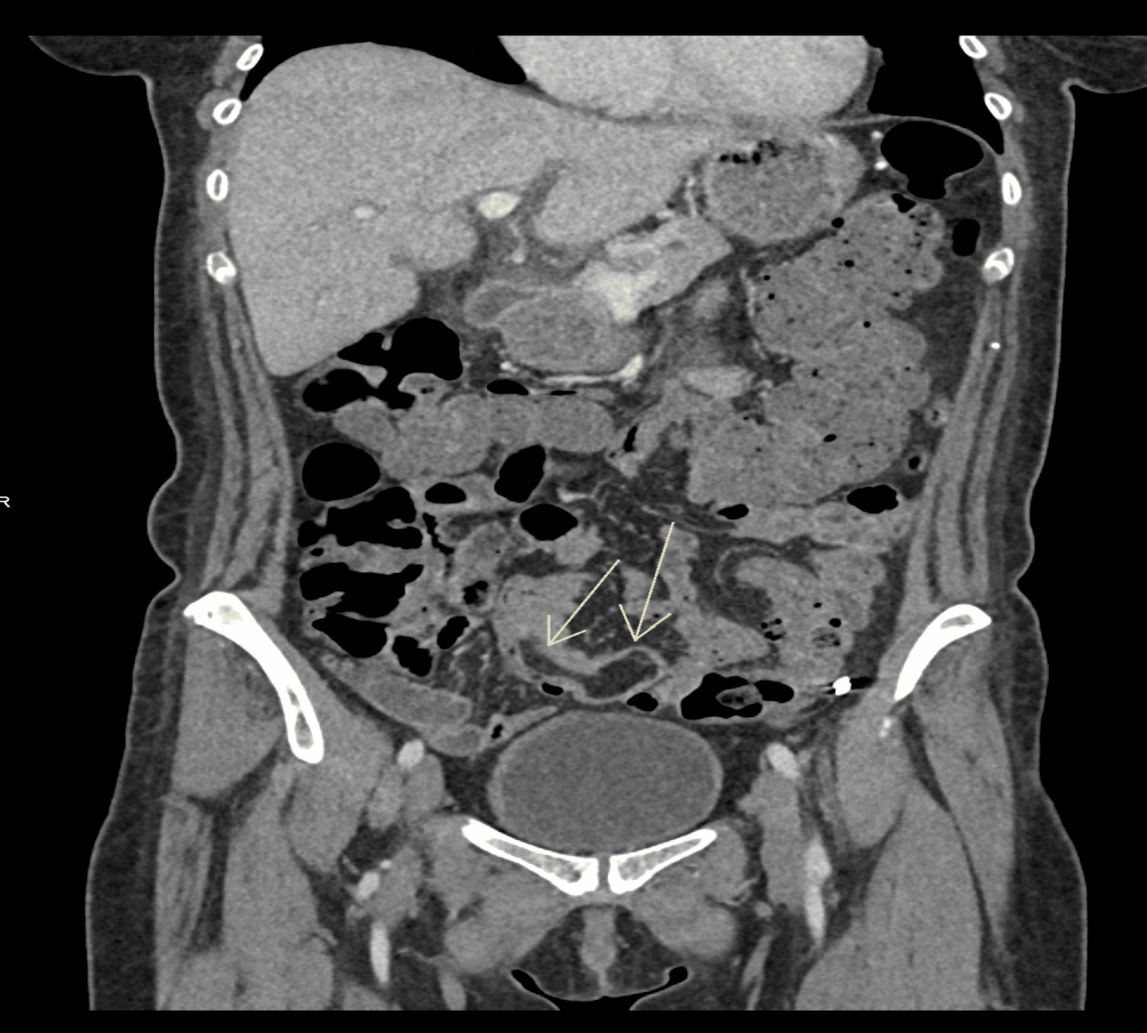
Coronal view of the polyp shown on CT scan abdomen with contrast
